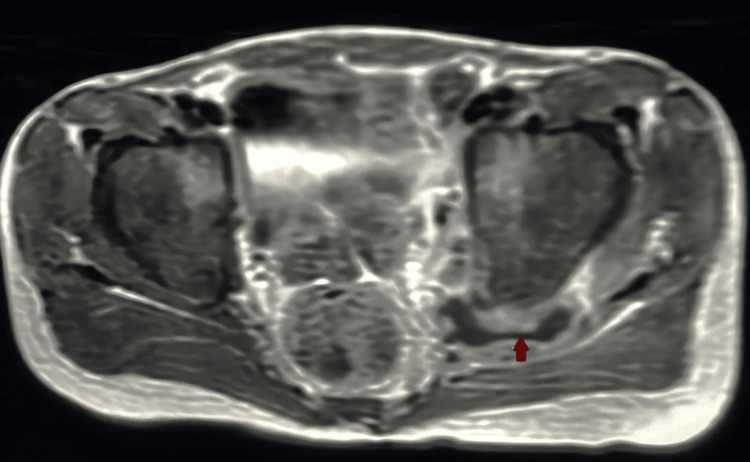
MRI showing enlarged left side pelvic floor muscles with small irregular abscess
